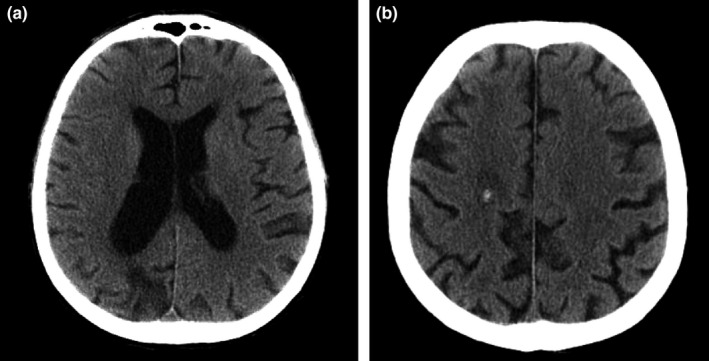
A and B, Cerebral computed tomography of September 2009 identifying the persistence of a discreetly dilated aspect of the lateral ventricles. Persistence of superior right parietal calcification and persistence of right parieto‐occipital cortico‐subcortical hypodensity is also observed, with a sequellar aspect. The aspect is in favor of the persistence of complete remission
